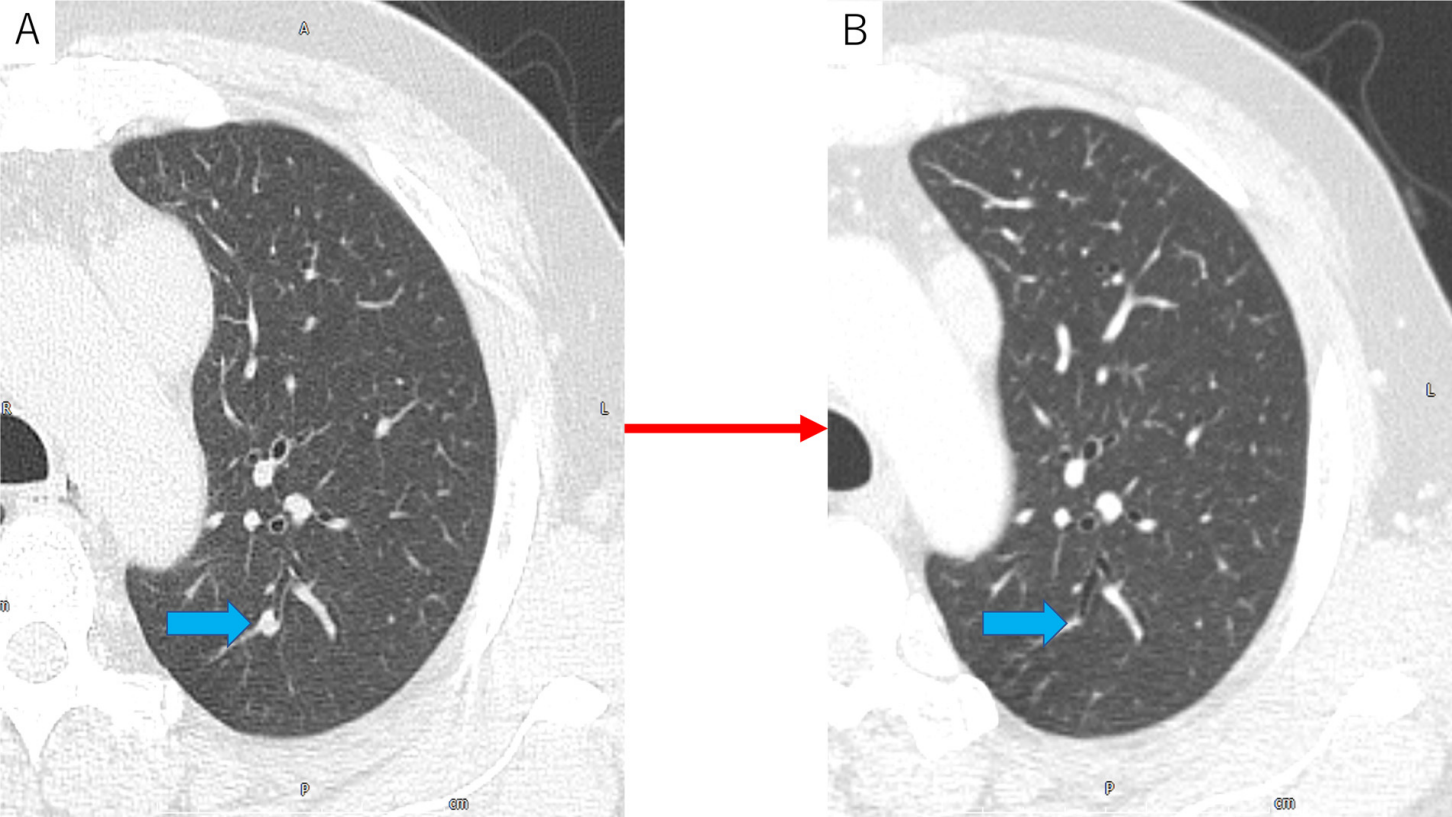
(A) CT examination at the patient's previous hospital revealed a pulmonary nodule of 4.5 mm in the left upper lobe (arrow). (B) Chest CT at the first presentation at our hospital revealed disappearance of the nodule (arrow).
